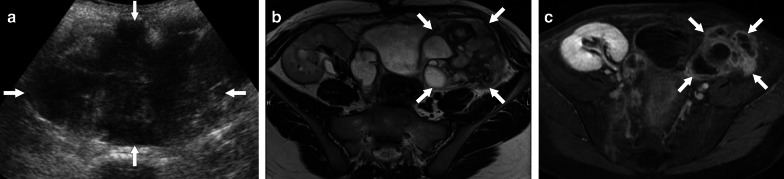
Pelvic inflammatory disease with tubo-ovarian abscess. A 23-year-old female with high fever, vaginal discharge and pain in her left lower quadrant and deep pelvis. Her medical history included chronic renal failure treated with transplantation. a Transabdominal sonography reveals large, complex-looking, mostly cystic left lower quadrant mass (arrows). b Axial T2W MR image shows a complex-looking mass with internal cystic components (arrows). c Axial postcontrast T1W image demonstrates intense contrast enhancement within the walls of the mass (arrows). Percutaneous aspiration produced pus, and biopsy showed intense inflammatory changes. Cultures grew *Escherichia coli*, and patient was placed on IV antibiotic treatment after aspiration
